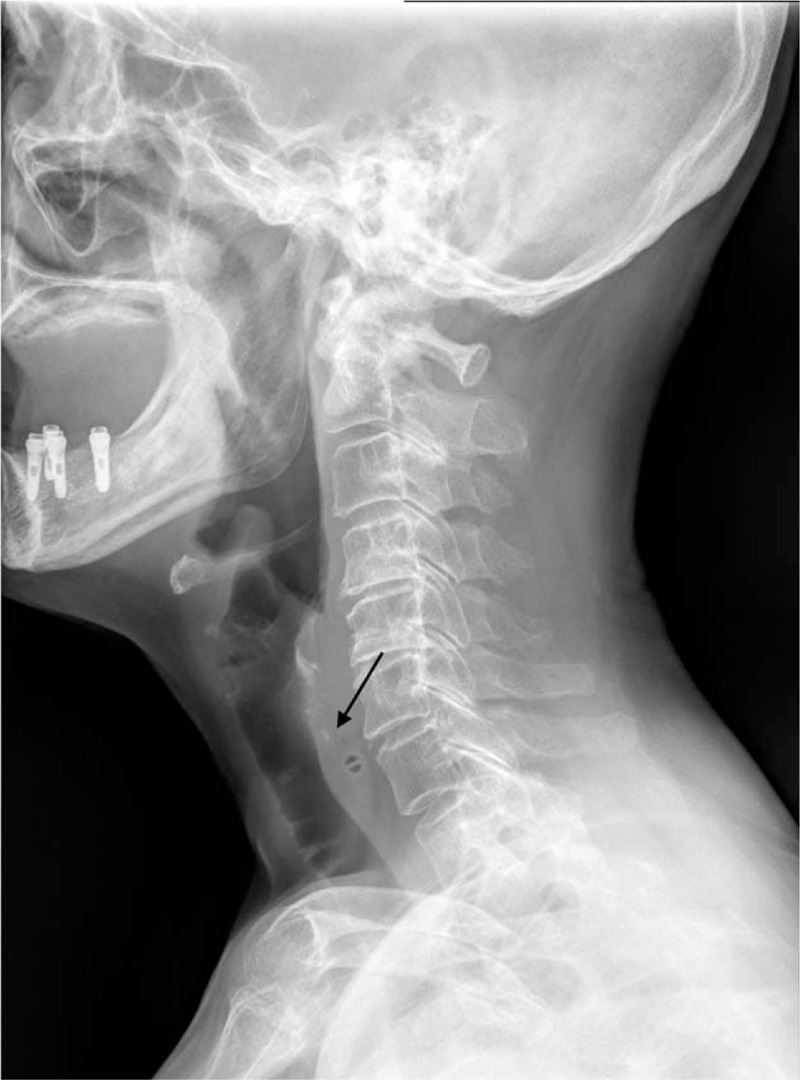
A 75-year-old woman (case 1) with complaint of odynophagia and neck pain that occurred 30 min after eating chicken porridge. Simple neck lateral radiography showing radiopaque material suspected as FB (black arrow). FB = foreign body.
